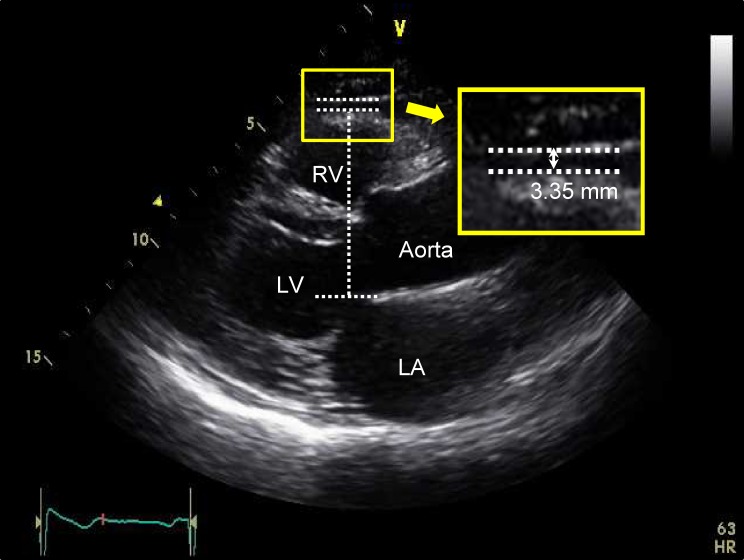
Method of measuring epicardial fat thickness. Epicardial fat thickness was measured perpendicularly on the free wall of the right ventricle from parasternal long axis view at end-systole. LA, left atrium; LV, left ventricle; RV, right ventricle.
